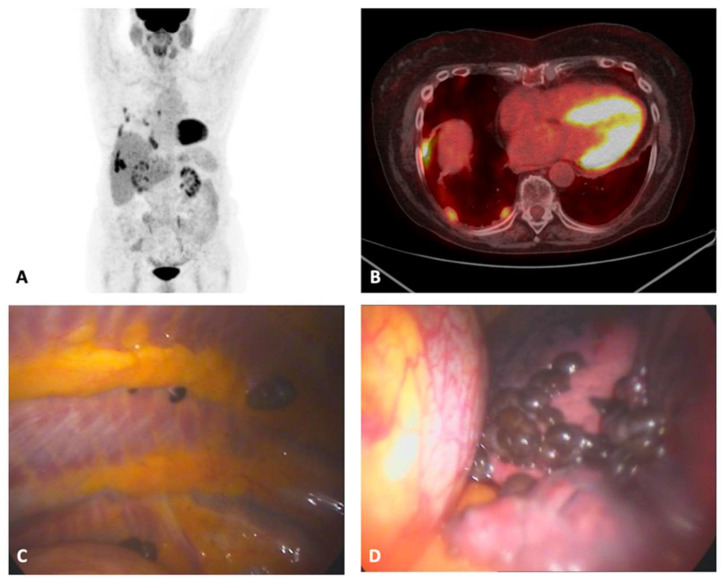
Case 4. (A,B) FDG-PET scans at presentation showing multiple right pleural thickenings with FDG uptake. (C,D) thoracoscopic view of the metastatic pleural plaques. FDG-PET, fluorodeoxyglucose positron emission tomography.
